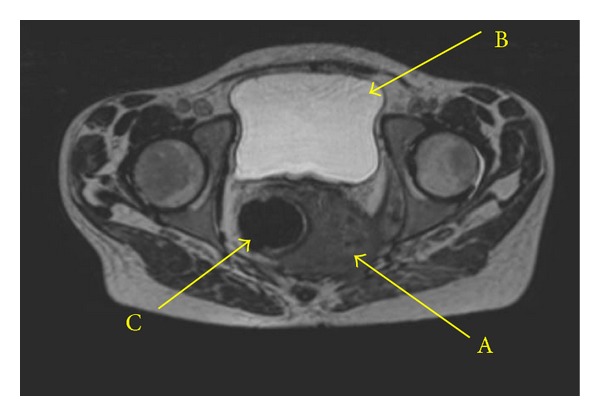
MRI scan of the small pelvic. A mass, originating from the perianal region, is expanding along the endopelvic fascia to dorsolateral with ingrowth in the left m. obturatoria and m. piriformis and ventral in the m. gluteus (Capitol A). No bladder ingrowth visible (Capitol B). The mass does have a direct relation with the wall of the lower rectum (Capitol C).
